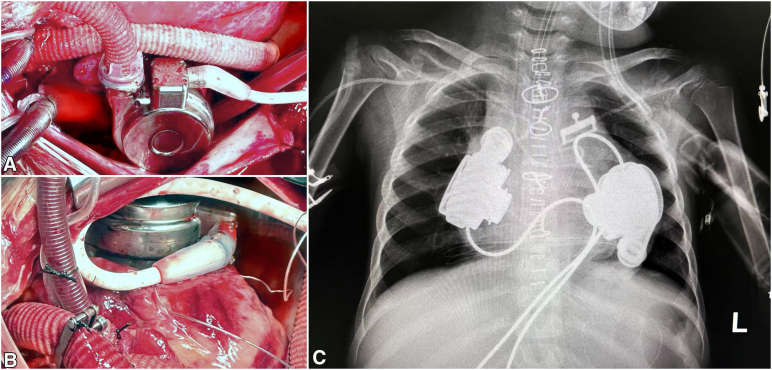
Implantation of LVAD and RVAD. A, An LVAD was implanted into the left ventricle and a custom-made flow restrictor was placed on the outflow graft of the RVAD. B, An RVAD was implanted in the right atrium with the LVAD outflow conduit being routed under the RVAD outflow conduit. C, Postoperative radiograph of the chest. *LVAD*, Left ventricular assist device; *RVAD*, right ventricular assist device.
